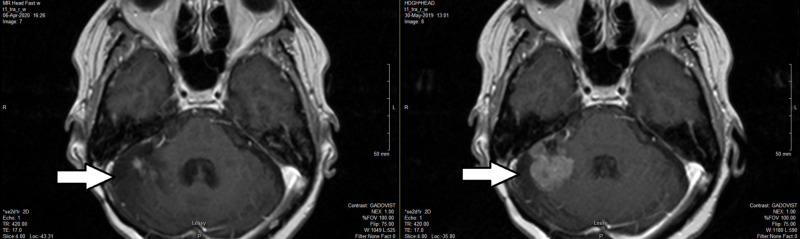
Brain MRI eight months after HSRT (left) versus two months before HSRT (right) HSRT, hypofractionated stereotactic radiotherapy
